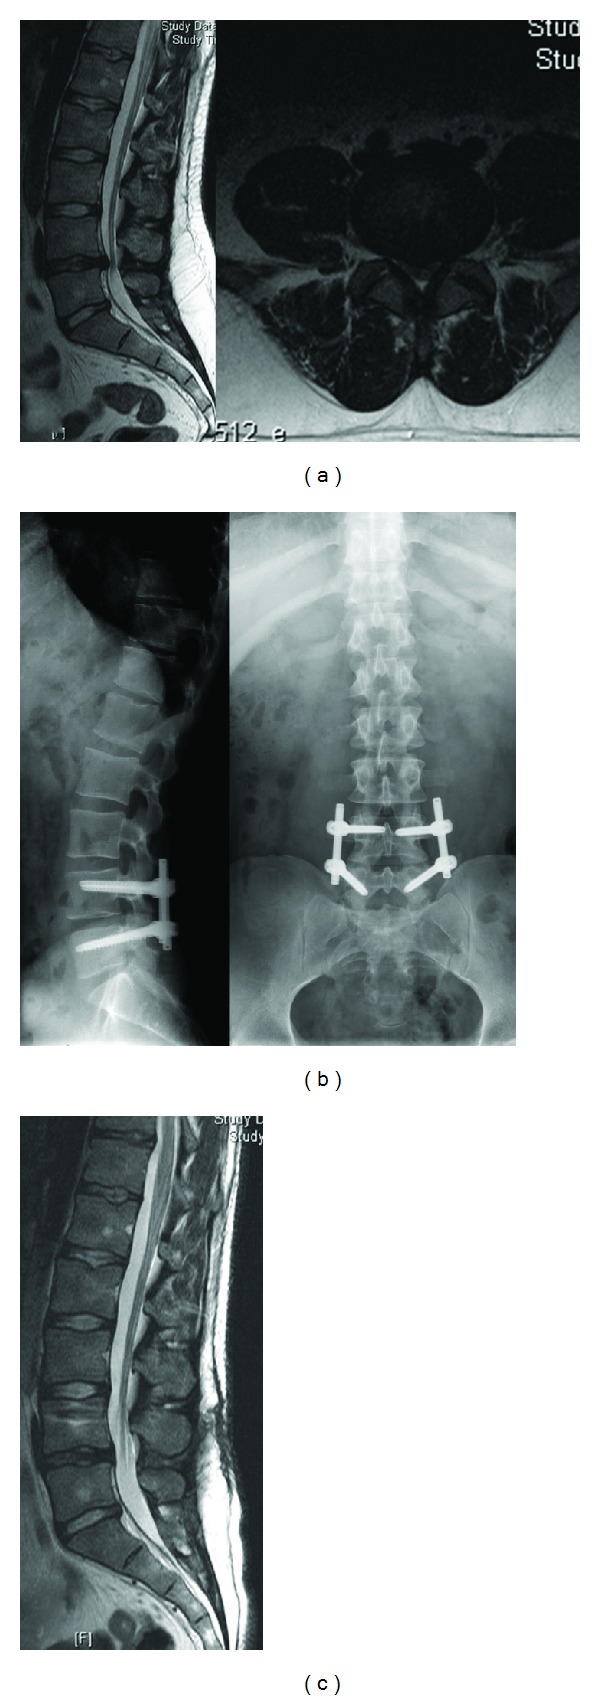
24-year-old male university student, with acute attacks of low back pain (2–4 times per year). He experienced right leg pain in a sciatalgia form, accompanied by severe low back pain in the last attack. In his neurological examination, he had L5 hypoesthesia but no motor deficit. (a) T2 weighted MRI scans show l4-5 disc herniation; (b) cosmic was applied; (c) the patient was in very good condition, and T2 weighted MRI scan shows a nearly normal appearance after one year.
